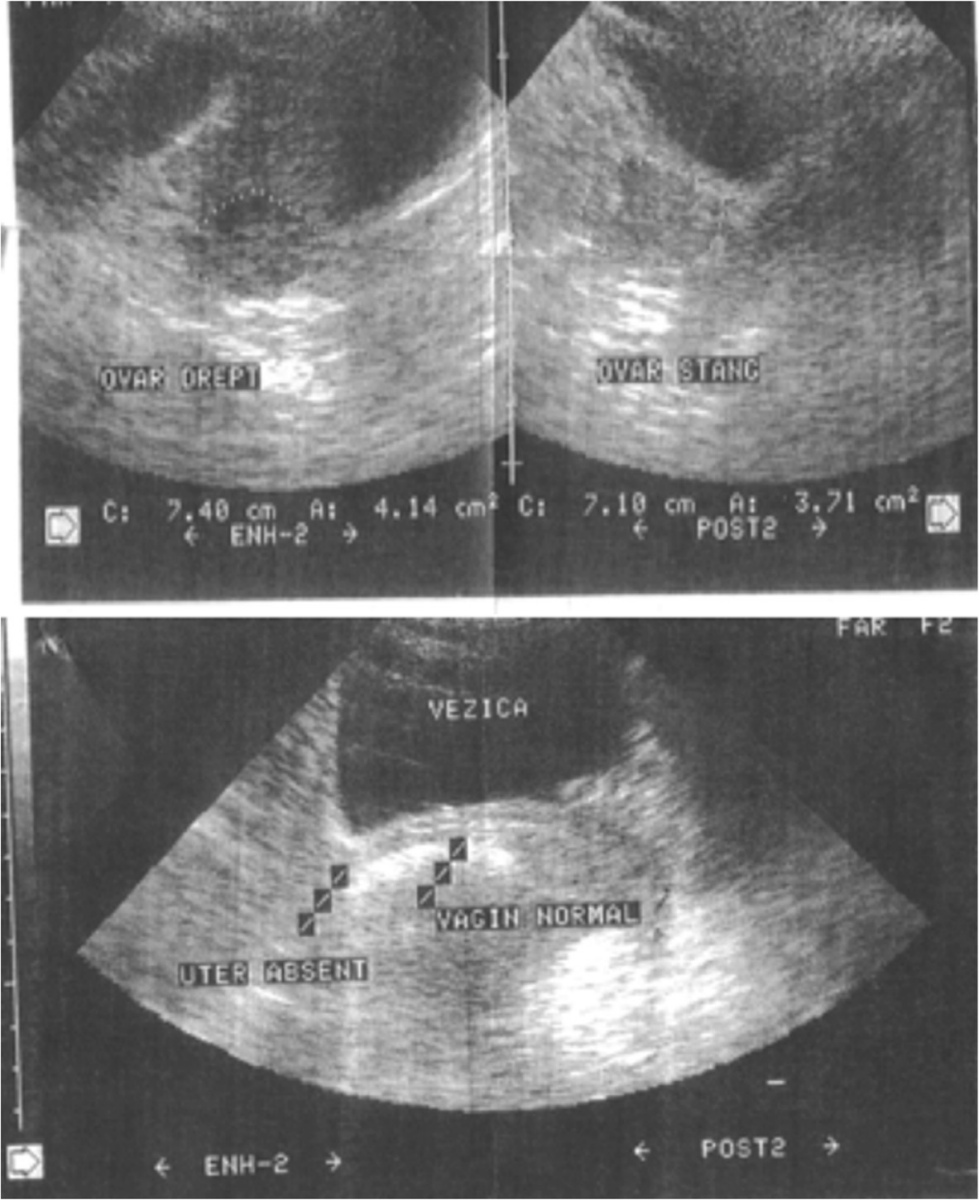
Pelvic ultrasonography images showing absence of the uterus, and the presence of both ovaries.
